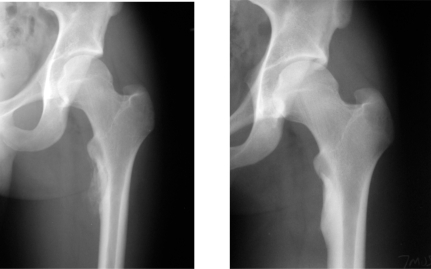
AP radiograph of femur of 23 year old male with an aneurysmal bone cyst involving the subtrochanteric region of the femur following resection and allograft packing. Left: incorporation at 6 weeks.  Right: consolidation at 3 months.
